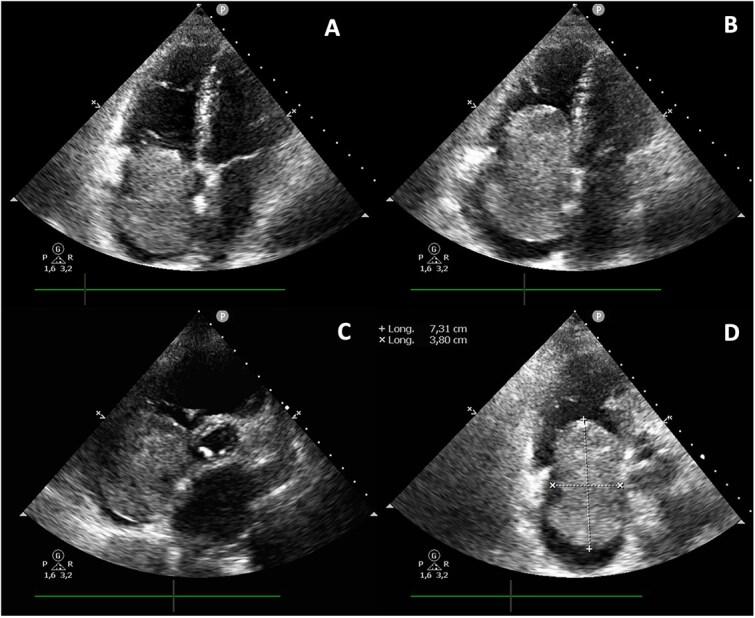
Echocardiographic evaluation revealing a large (73 × 38 mm), pedunculated, and hyperechogenic mass attached to the interatrial septum, occupying most of the right atrium and extending toward the tricuspid valve, visualized in the transthoracic apical 4-chamber view (A, B), parasternal short-axis view (C), and a modified apical 4-chamber view focused on the right chambers (D).
